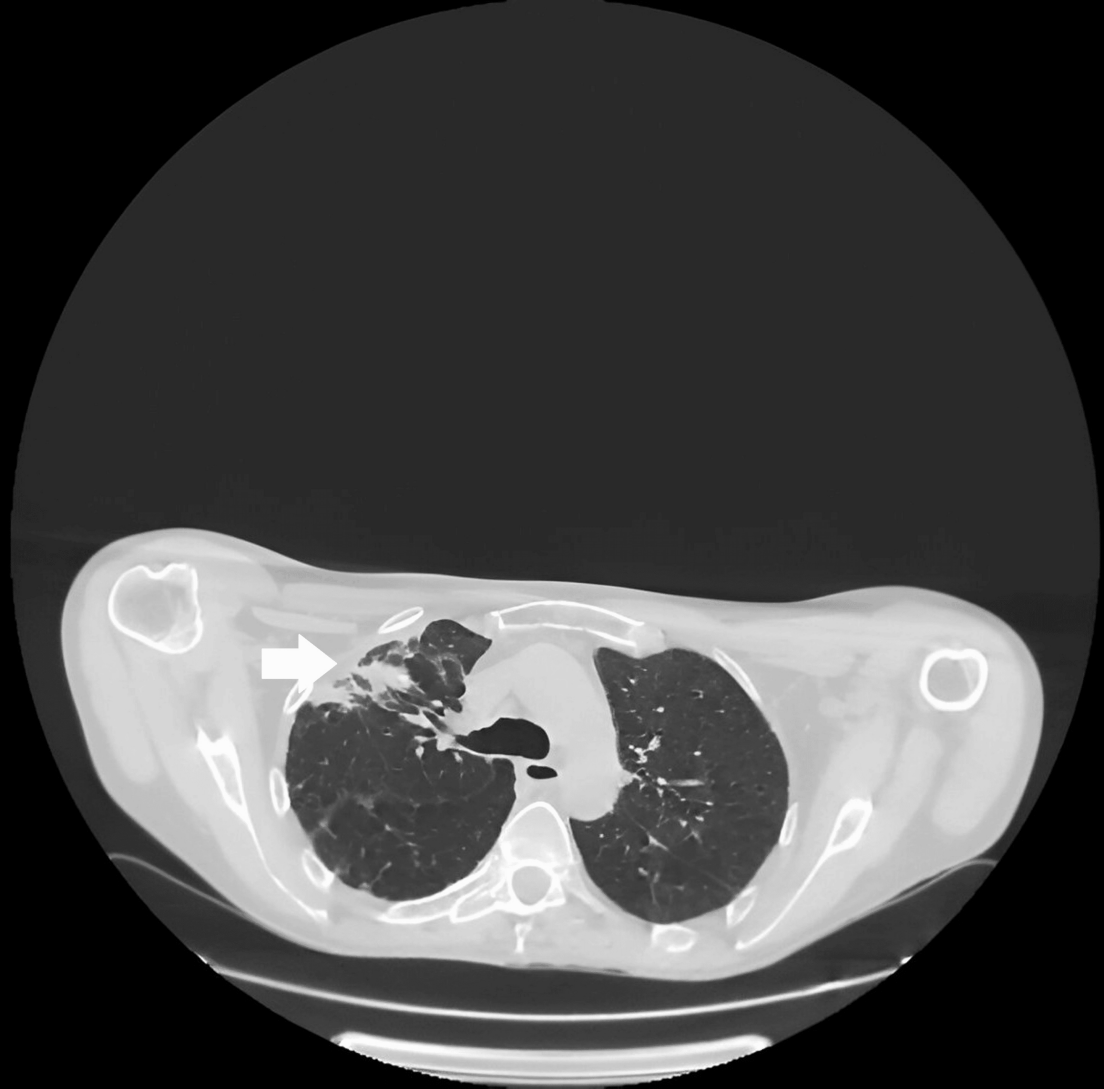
Thoracic CT scans revealing consolidation of the right lung apex with randomly distributed micro-nodules
